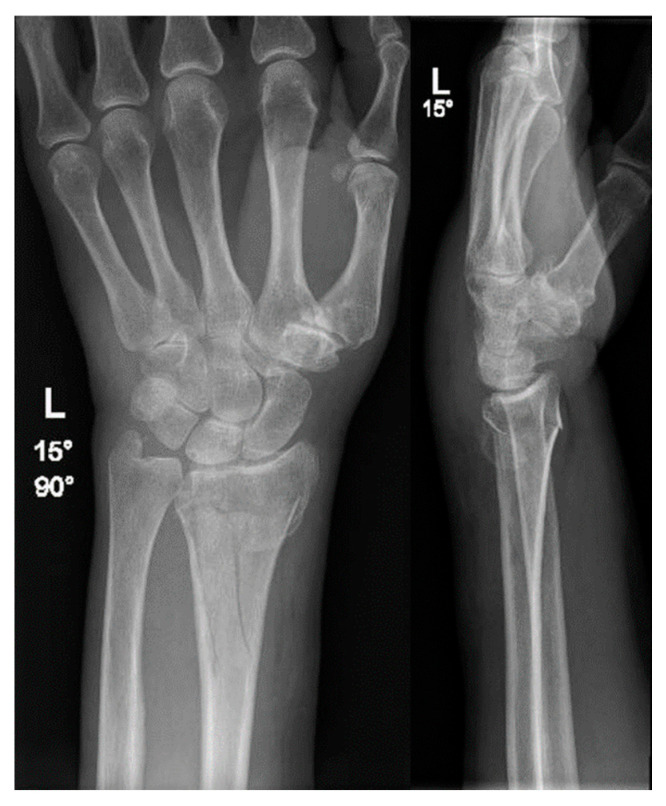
X-ray image in two planes of the initial fracture of the distal radius 4 years prior.
